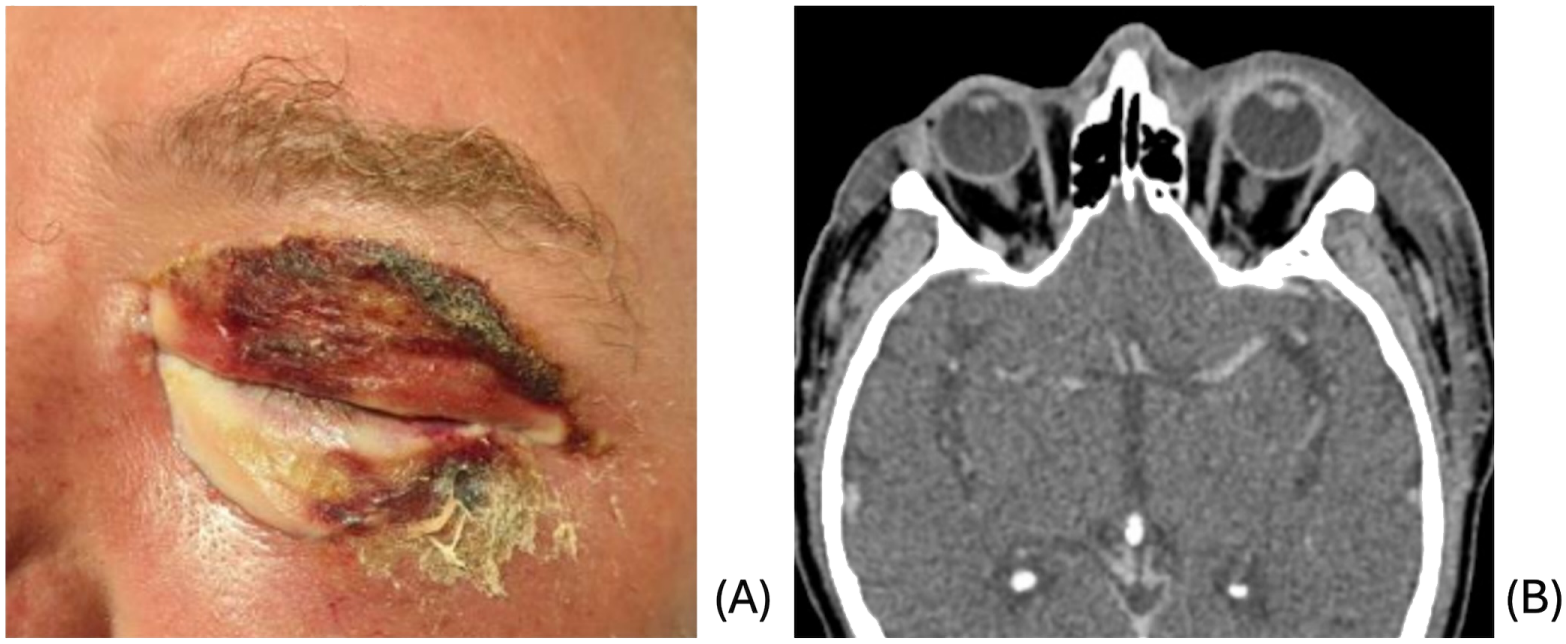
(A) Initial clinical presentation demonstrating periorbital edema and erythema with pale lower lid and scabbing of upper lid. (B) CT demonstrating extensive subcutaneous edema of the left face and preseptal cellulitis.
